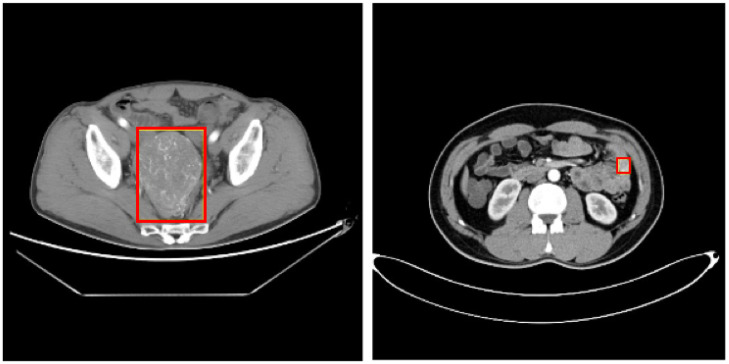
CT image of SIST (the red surrounding box is the focus area).
